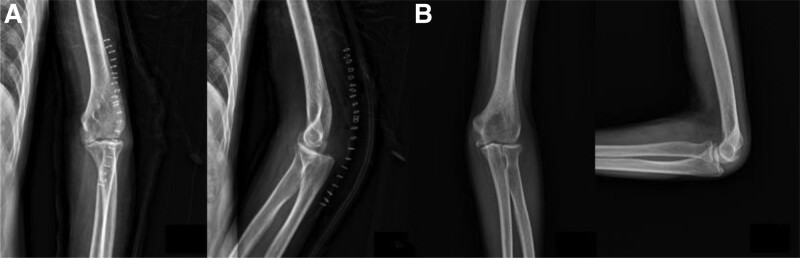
(A) Immediate postoperative X-ray. (B) After 3 mo follow-up X-ray.
